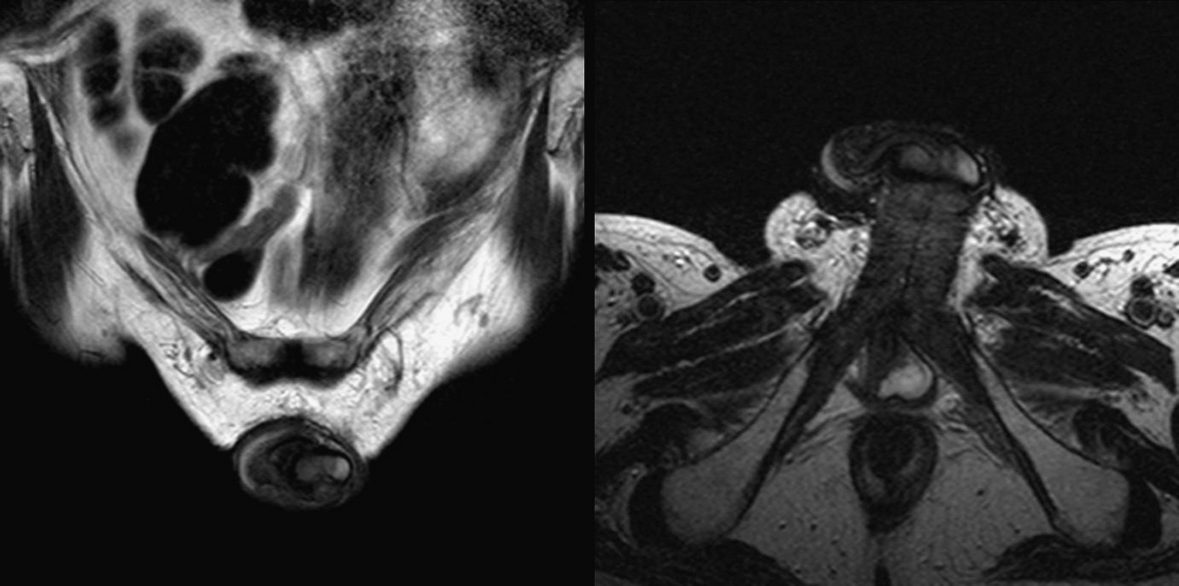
Magnetic resonance images of the pelvis. In this high-intensity area on T2, a marginal obscuration of the Buck’s fascia appears thickened around the left corpus cavernosum. (a) Coronal image. (b) Transverse image.
